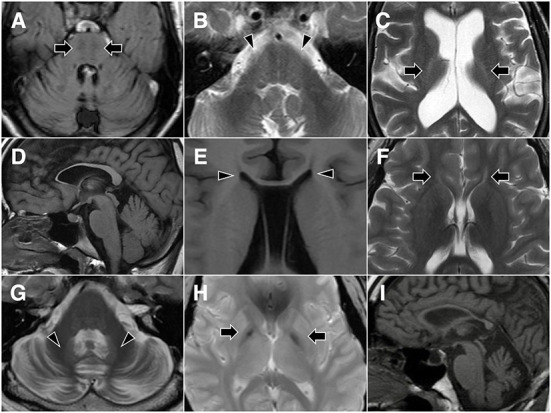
Representative brain MR images. Patient R2 shows horizontal hypointensity strips on FLAIR imaging (A, arrows), along with a hyperintense zone in the lateral pons on T2-weighted imaging (B, arrowheads) and a hyperintense peri-thalamic rim (C, arrows). Patient S13 shows thin corpus callosum (D) and a box-shaped calloso-caudate angle (E, arrowheads) on T1-weighted imaging, as well as hyperintense streaks in the forceps minor of the corpus callosum (“ears of the lynx” sign) on T2-weighted imaging (F, arrows). Patient D4 demonstrates cerebellar atrophy and T2 hyperintensity of the dentate nucleus (G, arrowheads), as well as T2 gradient-echo hypointensity of the medial globus pallidus (H, arrows). Midsagittal T1-weighted imaging of patient S2 shows thin corpus callosum and cerebellar atrophy (I).
